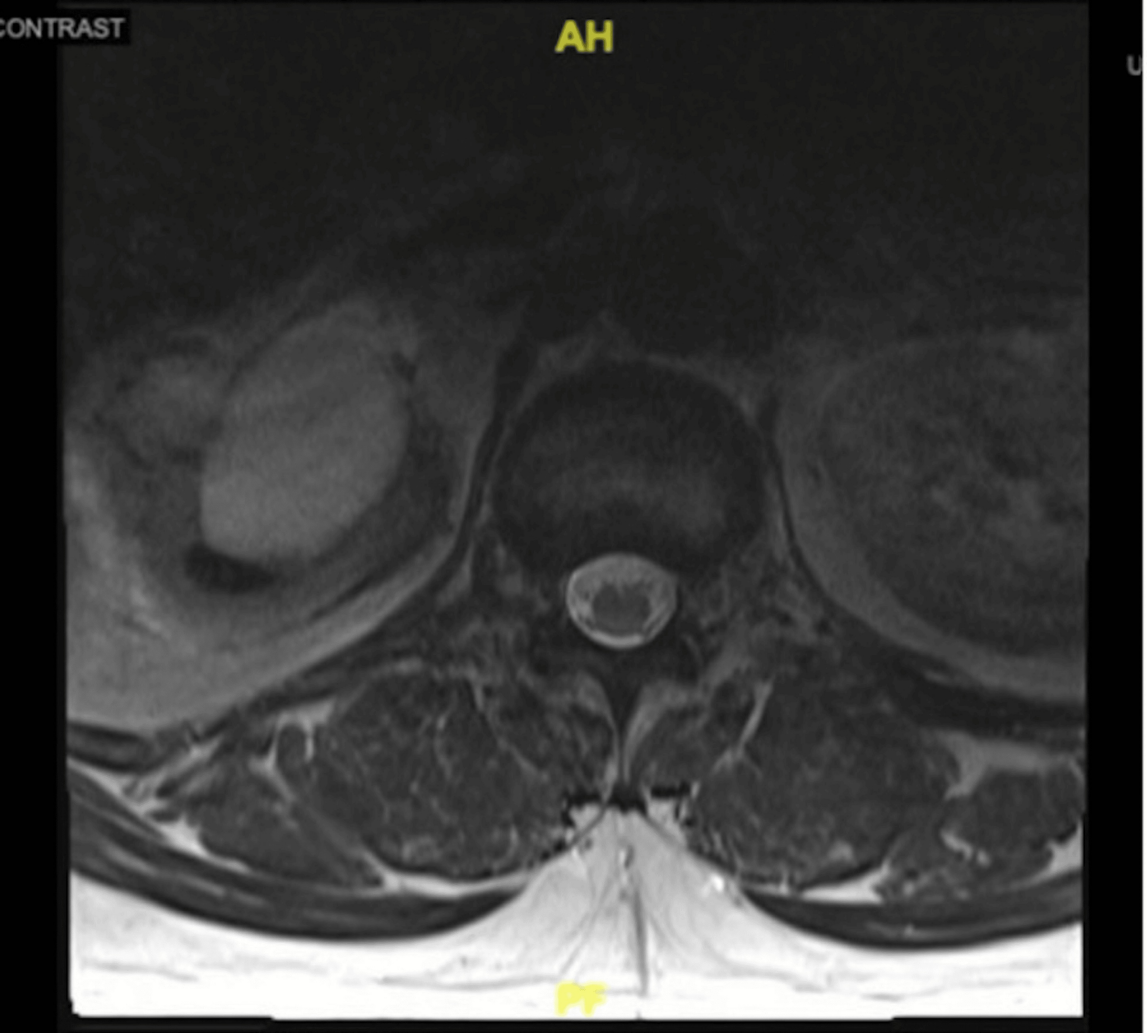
T2-weighted MRI showing an abnormal right kidney with hydronephrosis.
